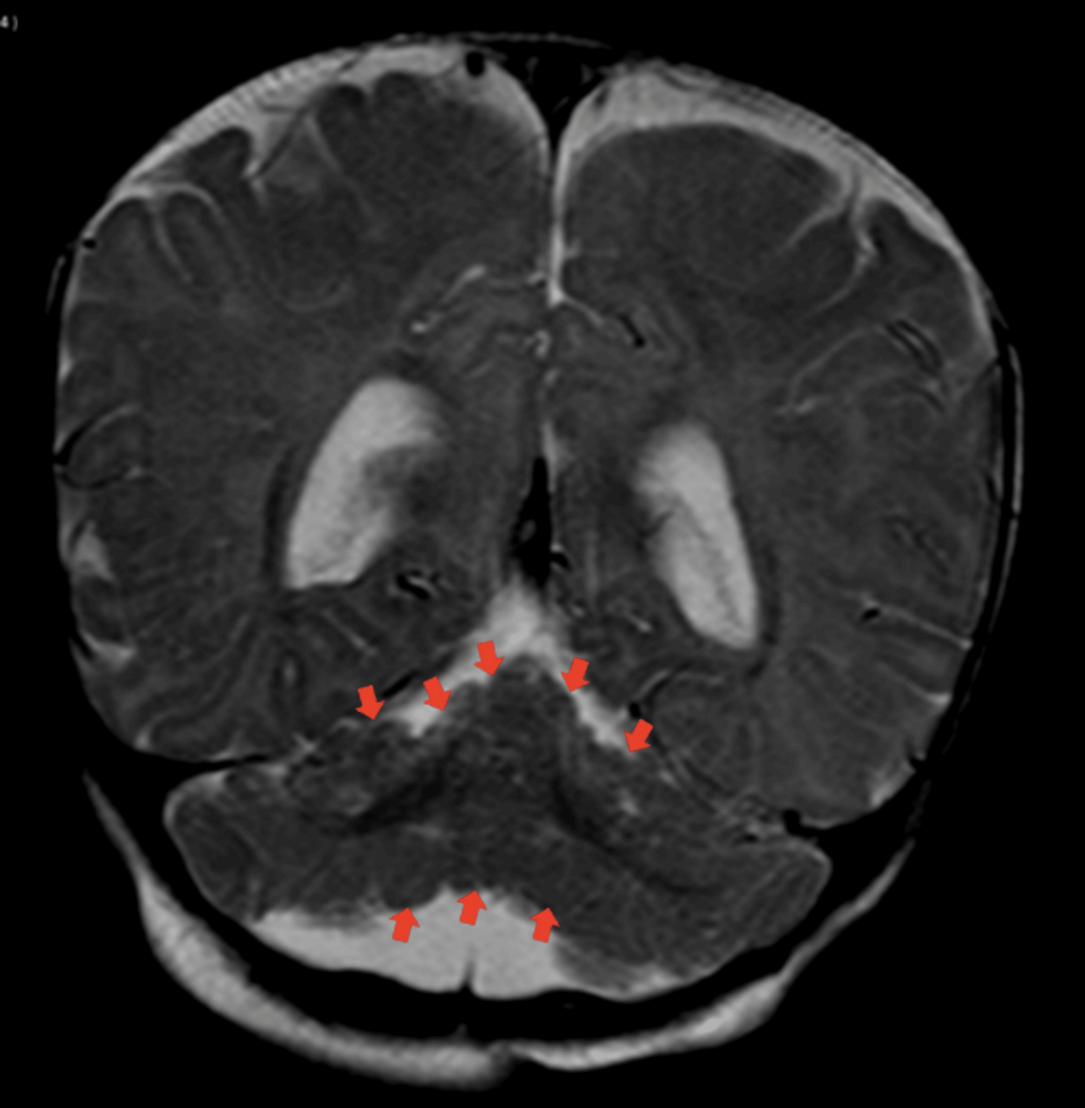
A coronal section of the T2 brain MRI scan of case 1 showing abnormal cerebellar foliation as labeled with the red arrows.
